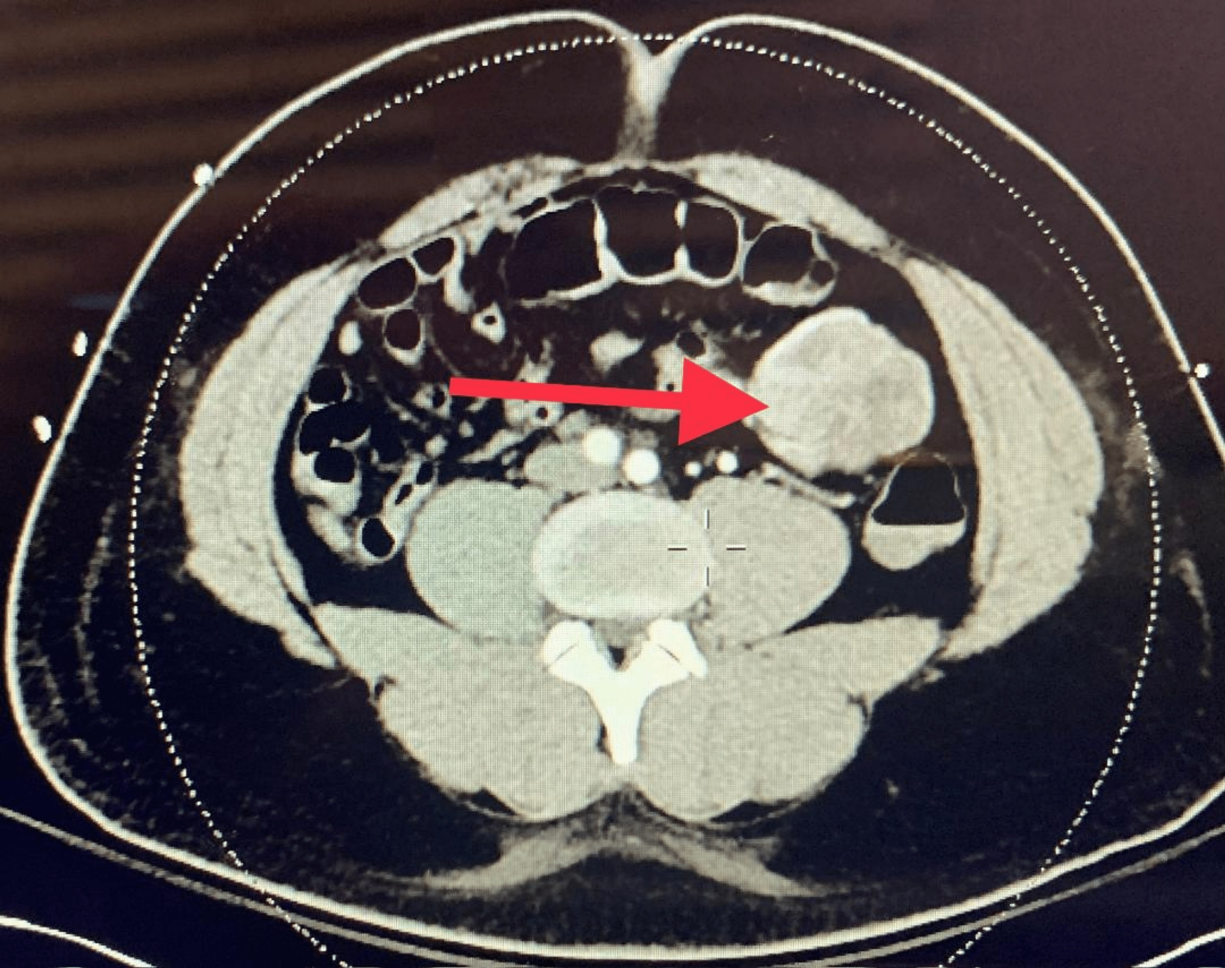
CT (axial) of the abdomen Soft tissue mass associated with small bowel loop in the left lower quadrant (red arrow) CT: computed tomography
